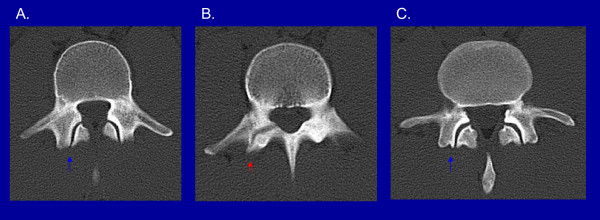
CT findings in a patient with pars interarticularis fracture: Normal facet joint below fracture (A), right L3 pars interarticularis fracture (B), normal facet joint above fracture (C). [red arrow points to the fracture and blue arrows point to normal facet joints].
